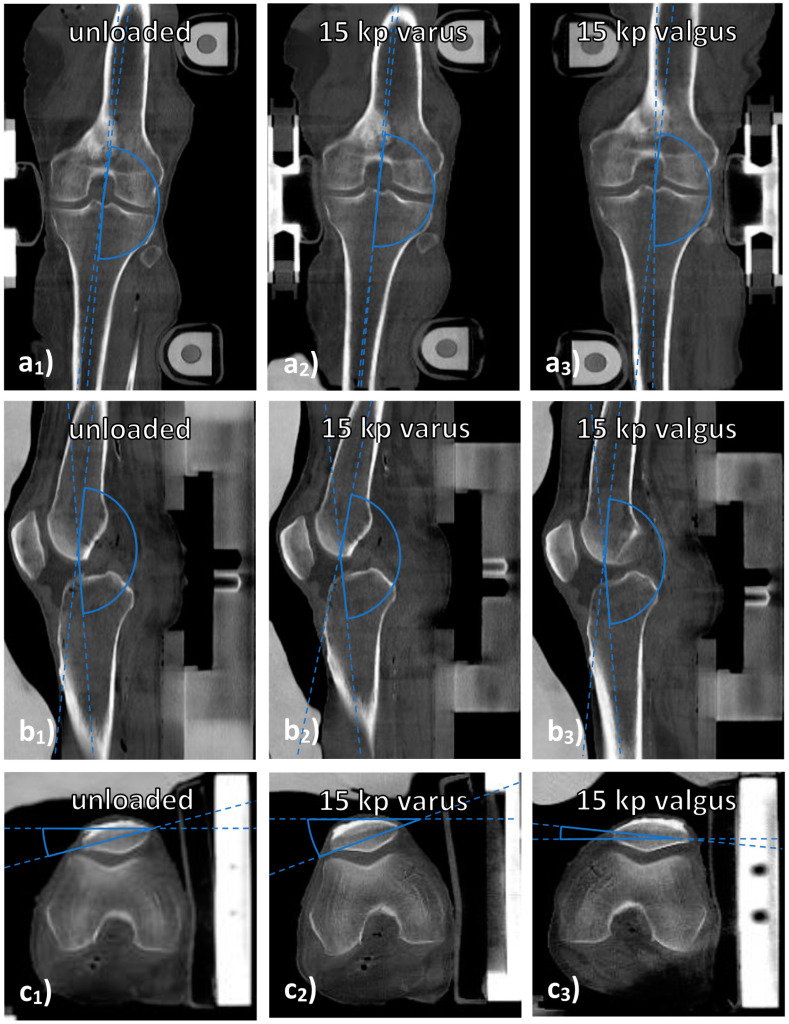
Measurements of joint alignment on CT images. Visualized are coronal (a), sagittal (b), and axial (c) reconstructions of a representative knee joint under various loading conditions, i.e., unloaded (a_1_–c_1_), under 15 kp varus loading (a_2_–c_2_) and under 15 kp valgus loading (a_3_–a_3_). “Joint deviation” was determined on mid-coronal images as the lateral angle between the femoral and tibial shafts (a). “Joint extension” was determined on mid-sagittal images as the popliteal angle between the femoral and tibial shafts (b). “Joint rotation” was determined on the axial images that demonstrated the largest diameter of the patella by relating the trans-patellar line (through the outer corners of the patella) to the horizontal line (c). By convention, external rotation was indicated by positive angles and internal rotation by negative angles.
